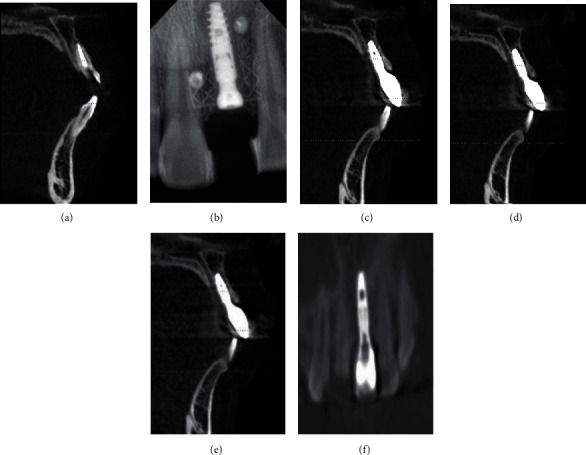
Radiographic findings. (a) Before implantation. (b) 6 months after implantation. (c) 6 months after restoration. (d) 1 year after restoration. (e) 2 years after restoration. (f) Osteogenic image around implant 2 years after restoration.
